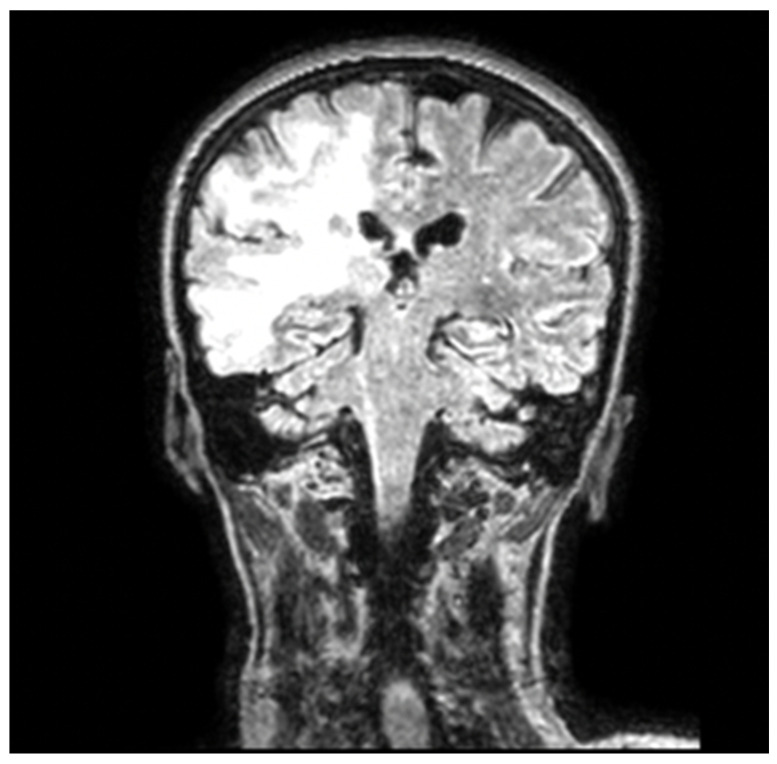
Coronal FLAIR brain MRI showing asymmetric hyperintense lesions in the left parieto-occipital subcortical and periventricular white matter, without mass effect or enhancement, which are findings suggestive of progressive multifocal leukoencephalopathy in an immunocompromised patient.
